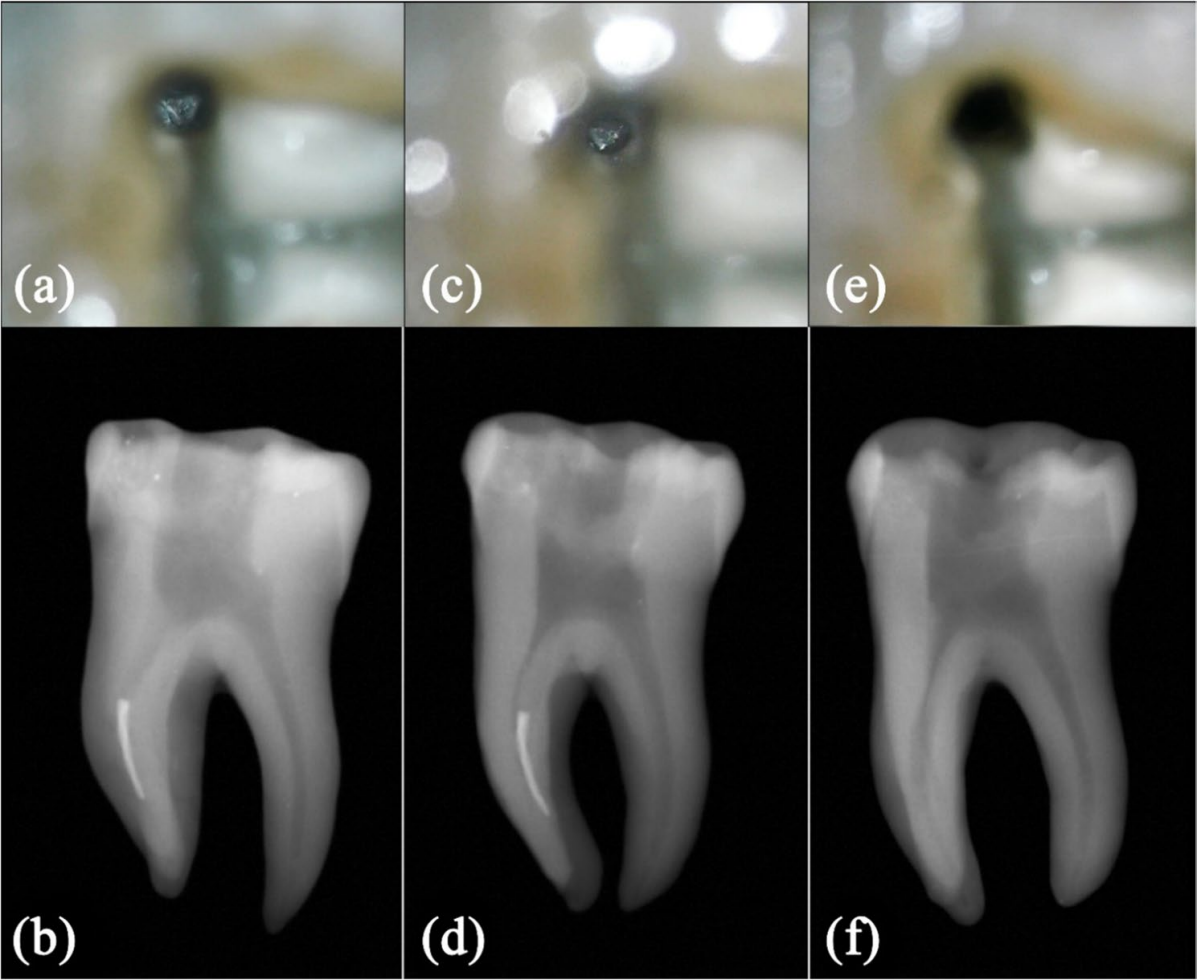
Modified staging platform (a) Clinical photograph of separated instrument within mesiobuccal canal under 11x magnification of DOM, (b) preoperative radiograph, (c)modified staging platform was created at 180° along inner canal wall, (d) intraoperative radiograph of the modified staging platform, (e) mesiobuccal canal after retrieval of instrument fragment. (f) postoperative radiograph to confirm complete removal of the instrument fragment without any retained metal fragments
